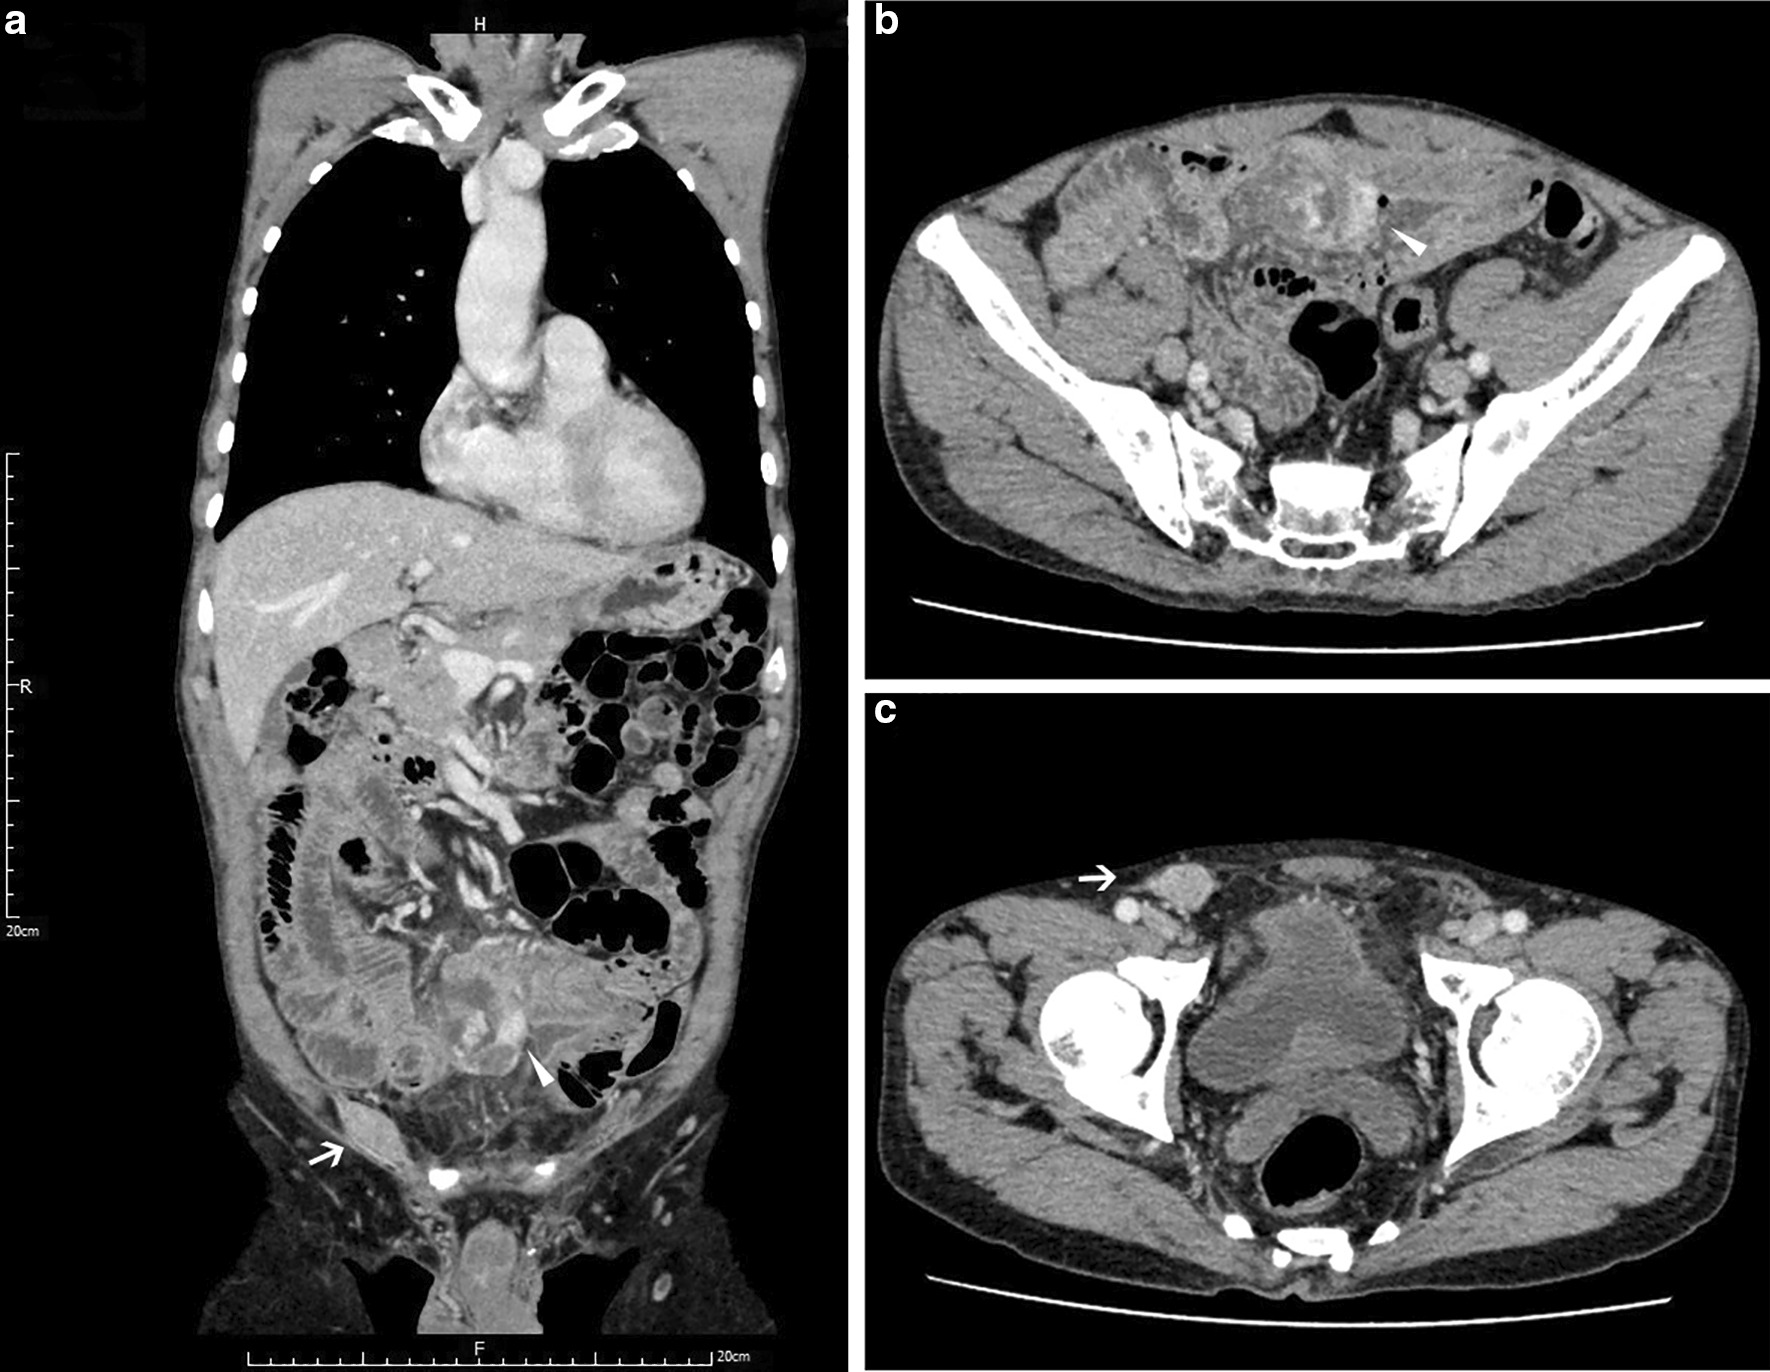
The radiological finding of the current case. CT images in the venous phase indicating a huge mass in the small intestine and an isolated inguinal mass in the right groin. Both masses were contrast-enhanced, without distinct lymph node metastasis in the abdomen. a Coronal plane rebuilt scan; b, c Horizontal plane scans; Arrows and triangle shapes indicating inguinal and intra-abdominal mass, respectively
